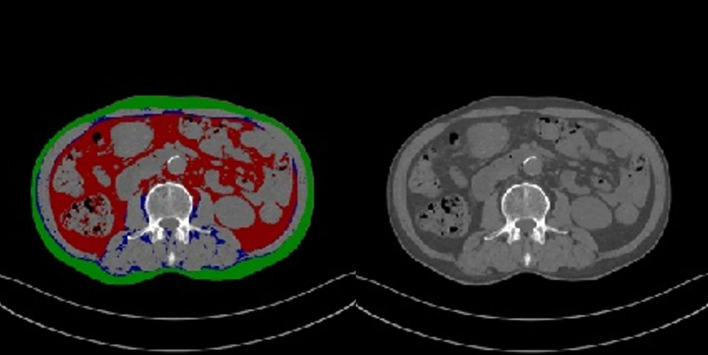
Example of CT-based body composition segmentation at the L3 level. Green, subcutaneous adipose tissue area; red, visceral adipose tissue area; blue, intermuscular adipose tissue area.
